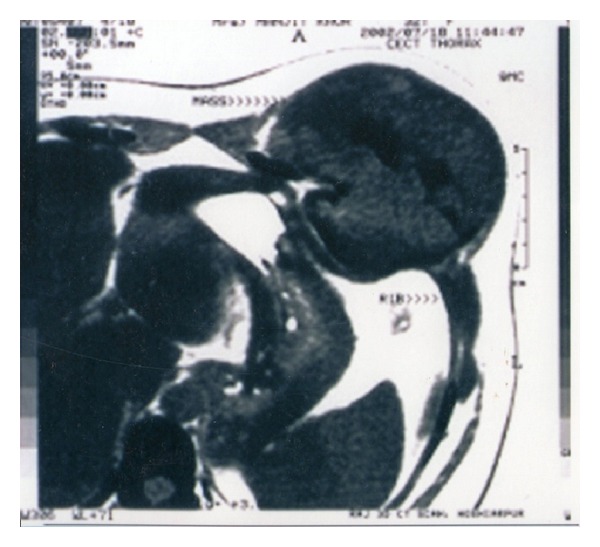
Giant cell tumor on CT scan of the chest. Computed tomography of the chest showing a large soft tissue mass arising anteriorly from the 7th rib, causing cortical destruction.
